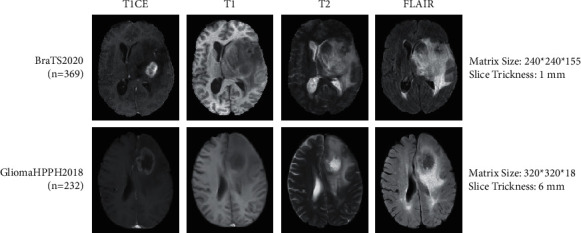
Image examples of 4-sequence MRI from BraTS2020 and GliomaHPPH2018 datasets.
